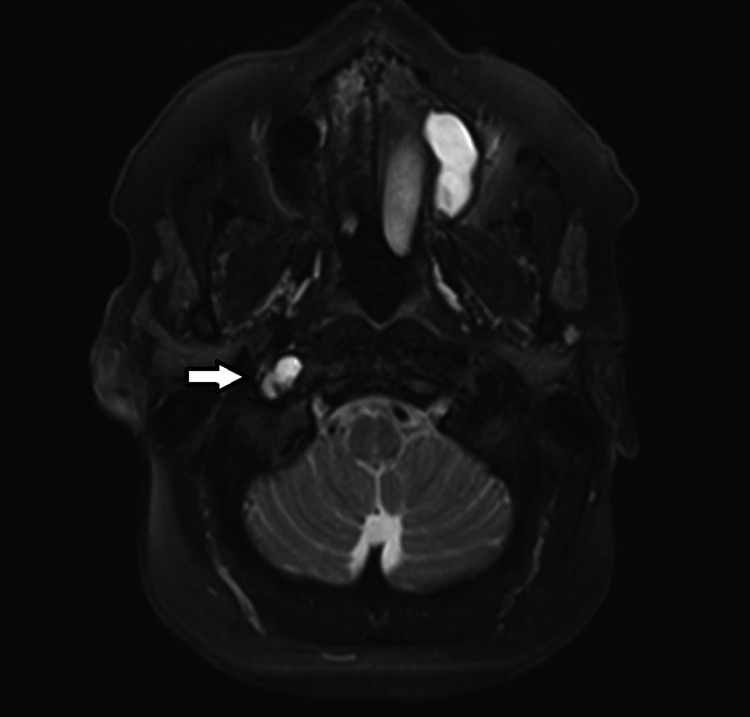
MRI of the soft tissue neck revealing a 1.5-cm cystic lesion inferior to the skull base in the post-styloid parapharyngeal space adjacent to the right AO joint. MRI: magnetic resonance imaging; AO: atlantooccipital
